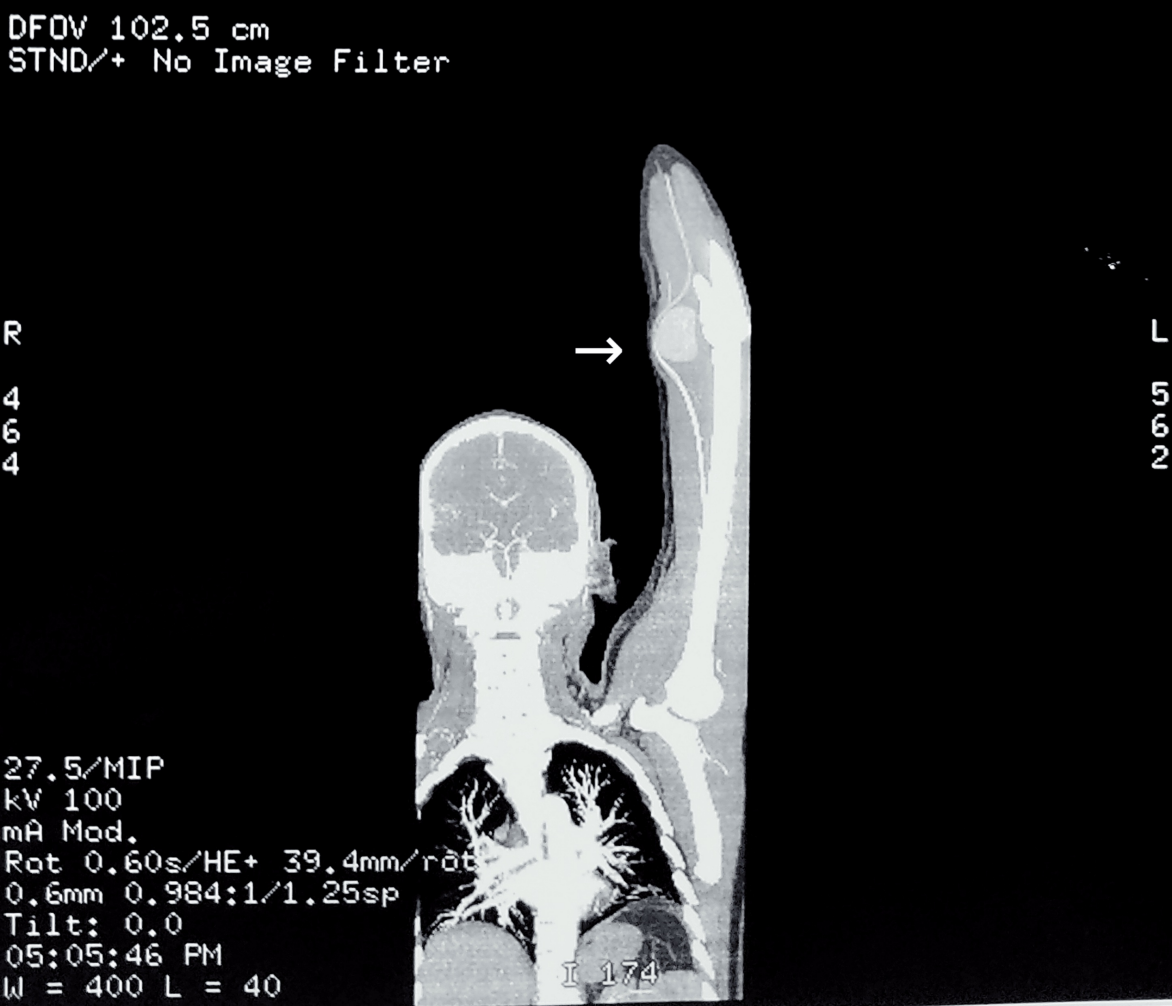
CT angiogram (coronal section) The image shows a well-defined aneurysmal outpouching, with complete contrast opacification, arising from the distal part of the brachial artery.
